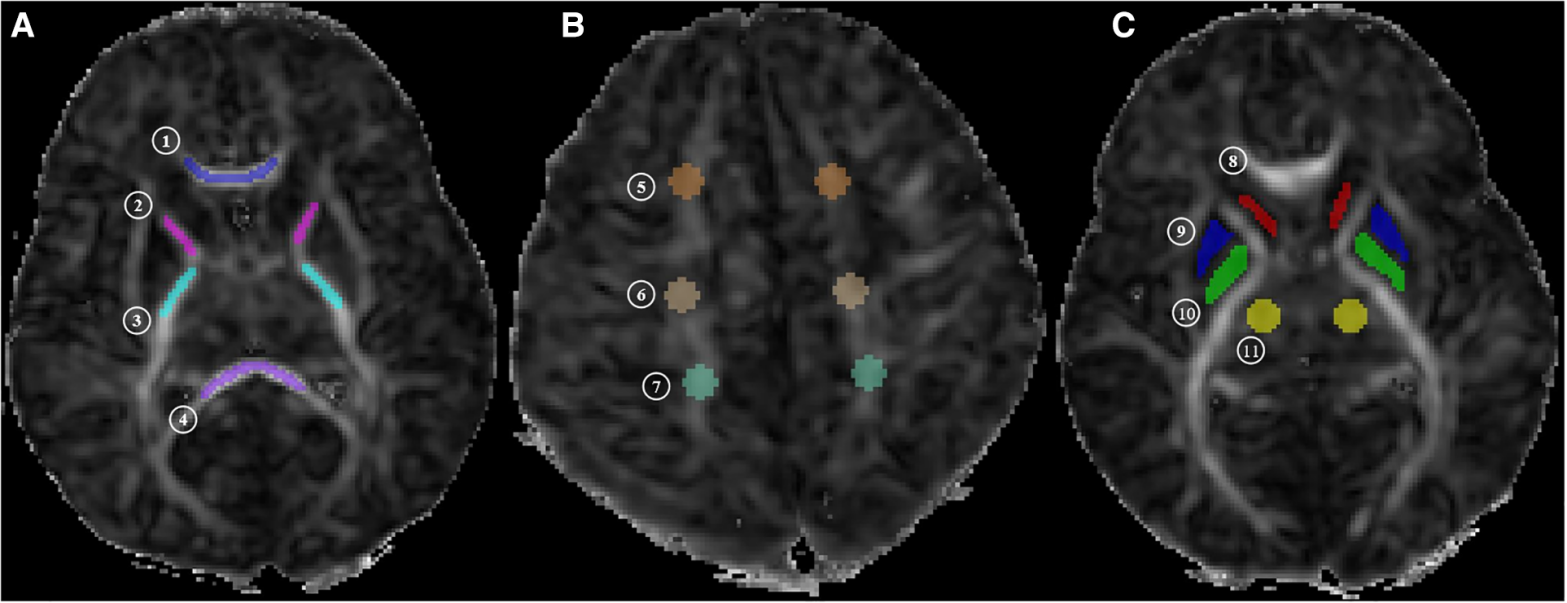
MR image shows. (A) Central white matter (level of the basal ganglia: ① genu of the corpus callosum [GCC], ② anterior limbs of internal capsule [ALIC], ③ posterior limbs of internal capsule [PLIC], and ④ splenium of the corpus callosum [SCC]); (B) peripheral white matter (level of the centrum semiovale: ⑤ frontal white matter [FWM], ⑥ central white matter [CWM] of the centrum semiovale level, and ⑦ parietal white matter [PWM]); (C) gray matter (level of the basal ganglia: ⑧ head of caudate nucleus [CN], ⑨ putamen [PUT], ⑩ globus pallidus [GP], and ⑪ thalamus [TH]). MK, mean kurtosis; Ka, axial kurtosis; Kr, radial kurtosis; FA, fractional anisotropy; MD, mean diffusion; Da, axial diffusion; Dr, radial diffusion.
